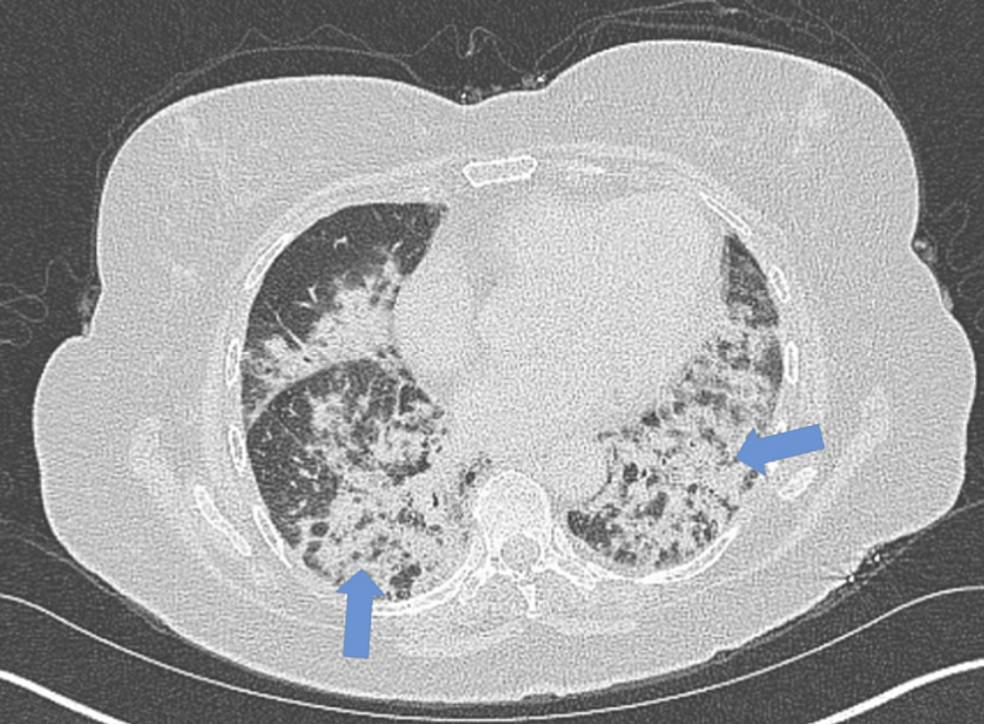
CT Thorax from outside the hospital upon initial symptom onset Extensive bilateral peribronchial consolidative changes, most prominent in the lower lobes, were noted (blue arrows).
